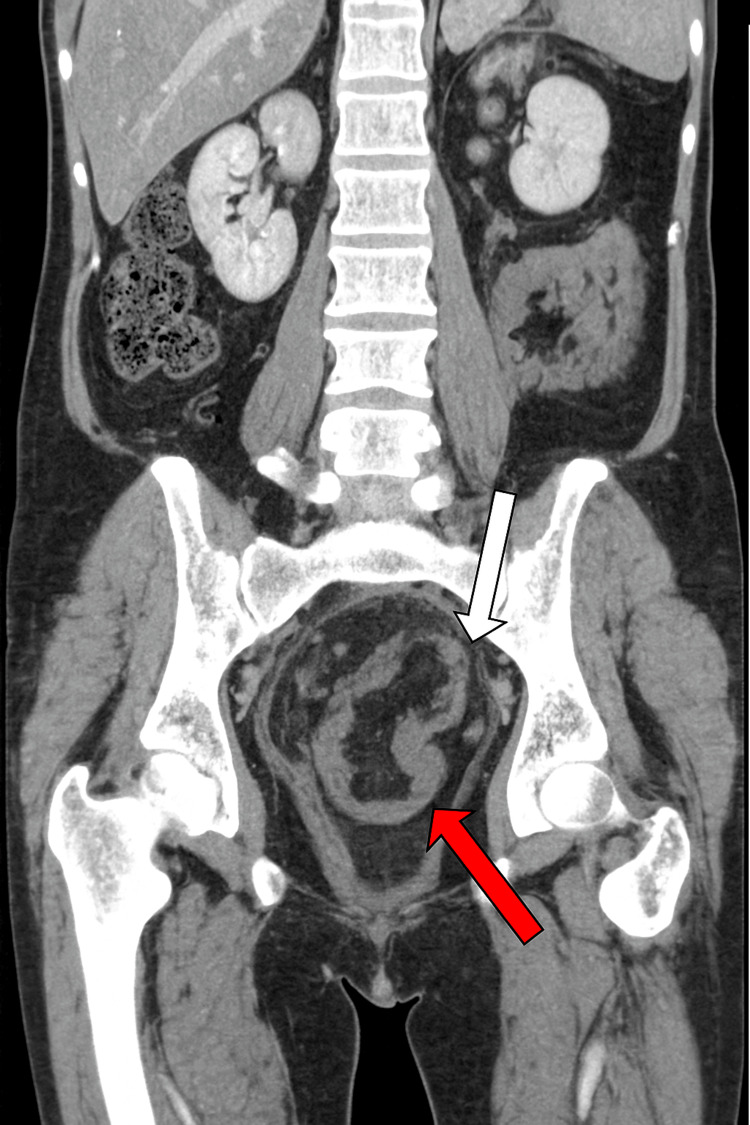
Contrast-enhanced CT (coronal view) showing rectosigmoid intussusception with the classic “target sign.” The red arrow highlights the intussusceptum (telescoping inner bowel), and the white arrow highlights the intussuscipiens (receiving outer bowel).
